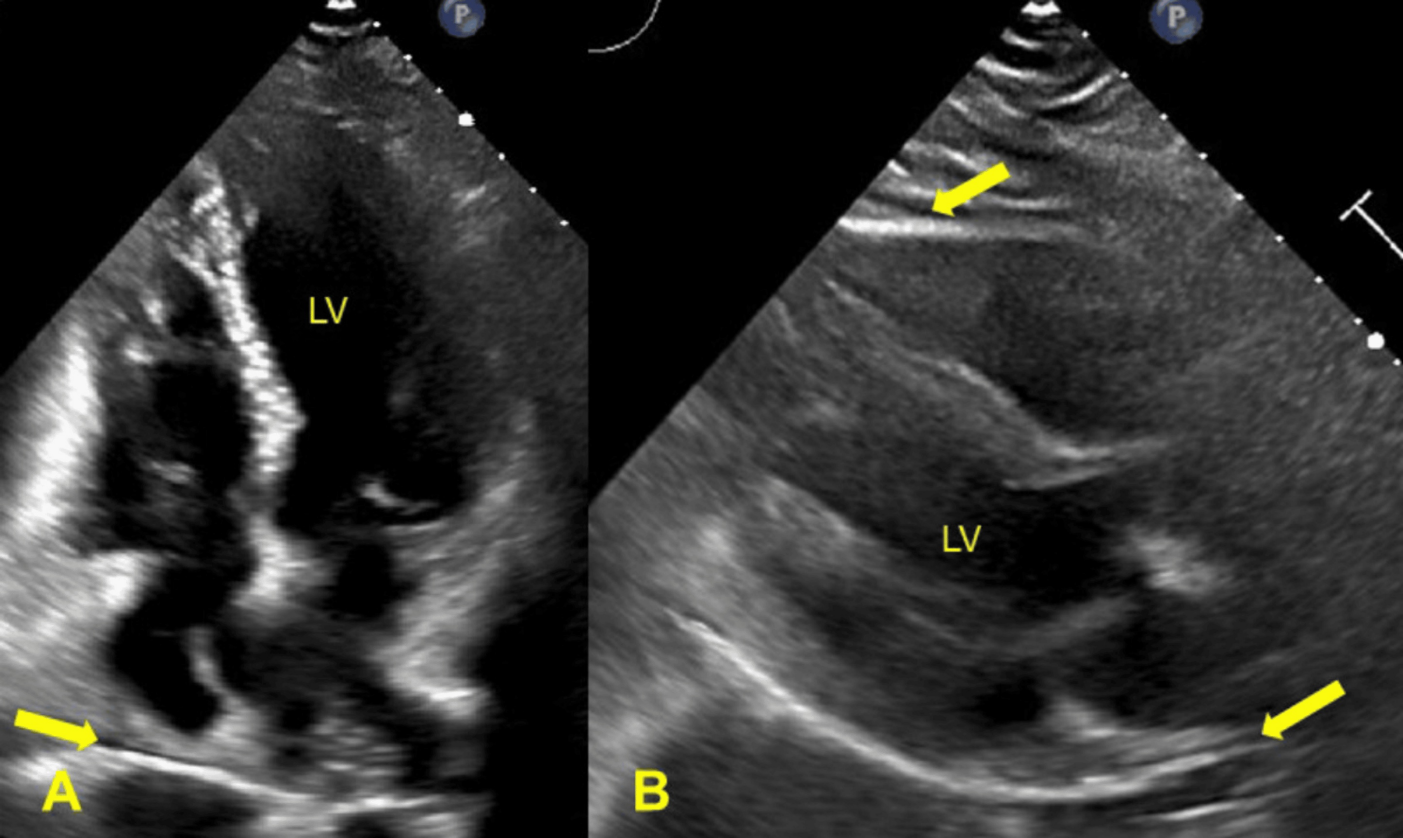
Transthoracic echocardiograms after treatment (day 68) Physiologic circumferential pericardial fluid is seen in (A) the apical four-chamber view (arrow) and B) the parasternal long-axis view (arrows) after pericardiocentesis and discontinuation of ibrutinib therapy. LV: left ventricle
